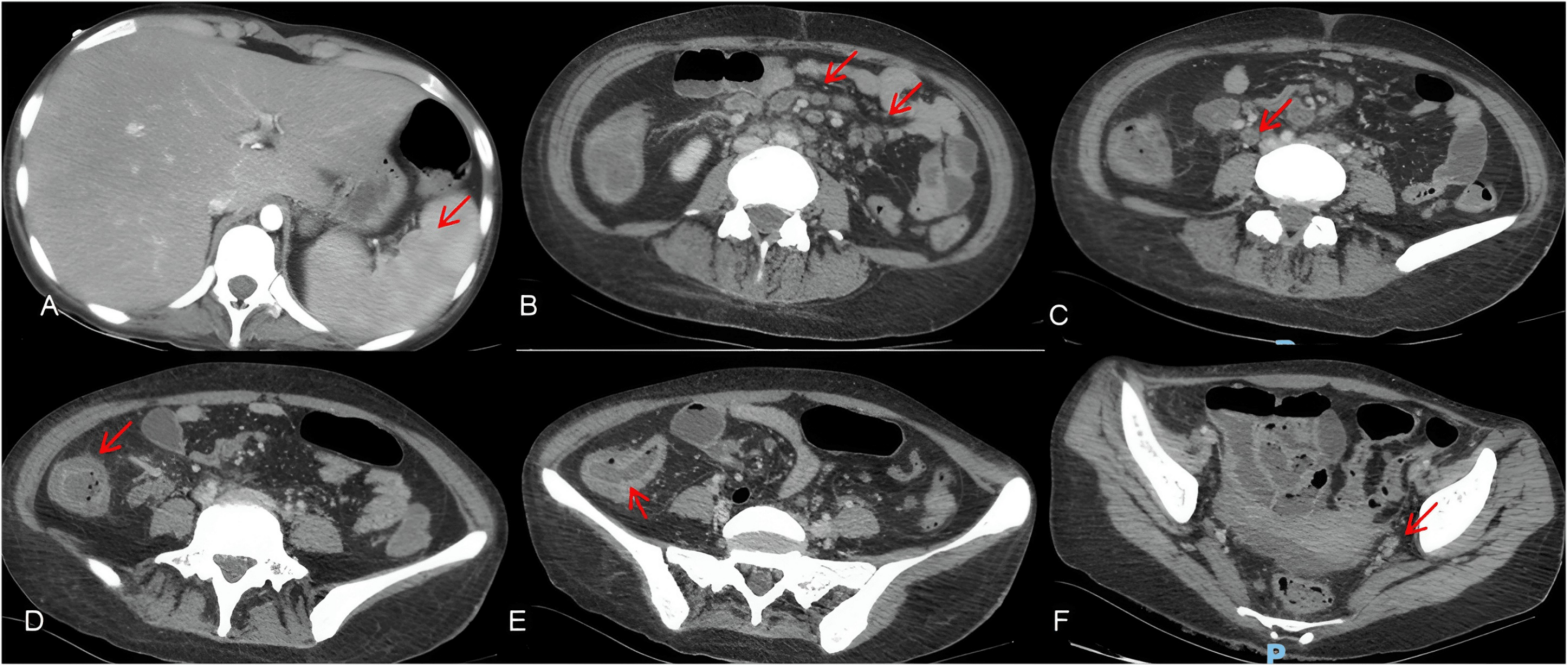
Patient's abdominal CT scan with contrast Abdominal CT scan with contrast showing liver and spleen enlargement with multiple hypoattenuating lesions on the splenic parenchyma, without contrast enhancement, suggestive of tuberculous microabscesses (red arrow in Figure 2A). Figures 2D and 2E exhibit intense cecal and ascending colon wall thickening by red arrows. Figures 2B, 2C, and 2F show multiple-sized, confluent lymphadenopathies in the hepatic hilum and the peripancreatic, periceliac, mesenteric, pericaval, iliac, and retroperitoneal chains, measuring up to 14 mm on on the smaller axis, most of them showing central hypodensity, suggestive of necrosis (thickening, red arrows).
